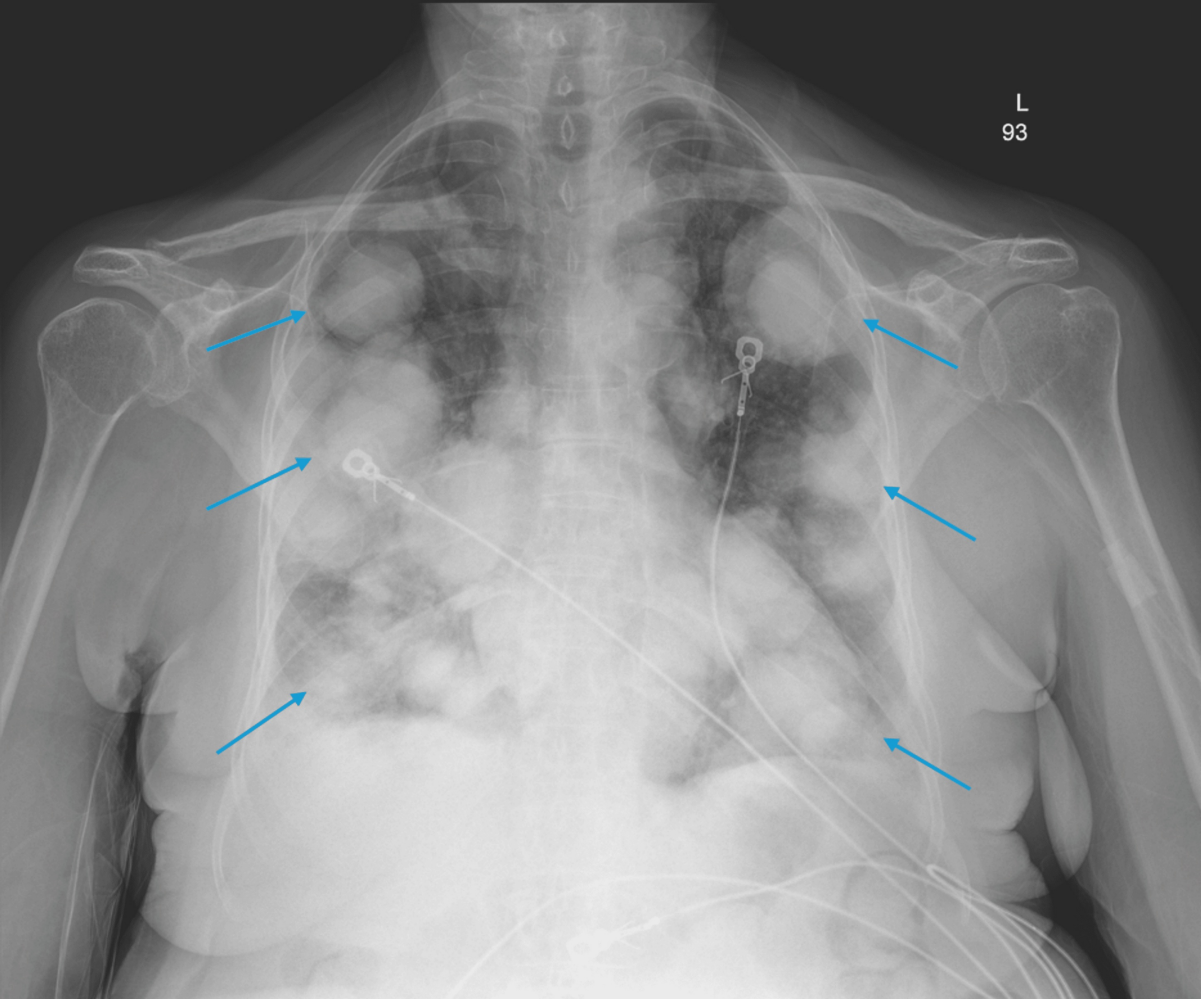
Chest X-ray on 4/20/2024 Mediastinum is midline. Normal cardiac silhouette. The blue arrow is pointing at numerous bilateral pulmonary masses. Bilateral costophrenic angles are blunted. No pneumothorax.
